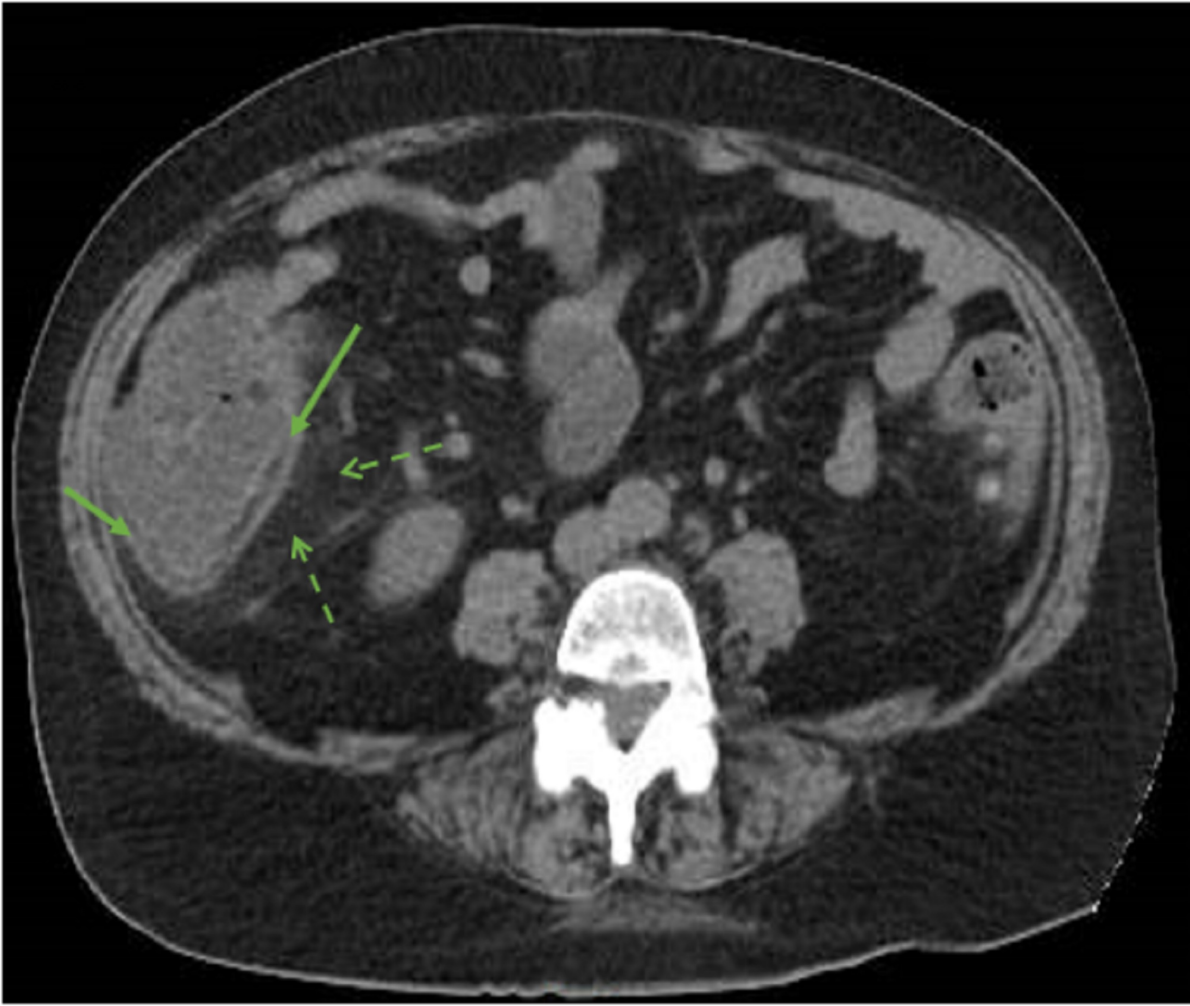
Inflammatory changes of cecum and ascending colon compatible with typhlitis. CT of the abdomen with intravenous contrast at the level of the cecum showing a circumferential wall thickening of the cecum and ascending colon (full arrows) with adjacent mesenteric fat stranding (dashed arrows) consistent with colitis.
